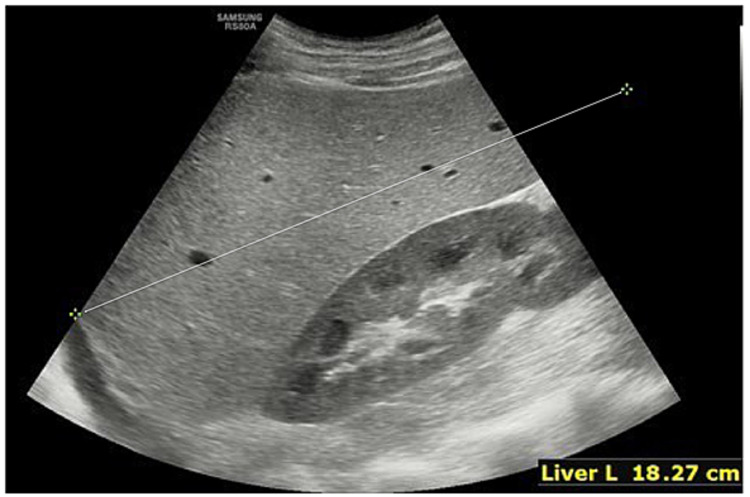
Ultrasound showing hepatomegaly.
